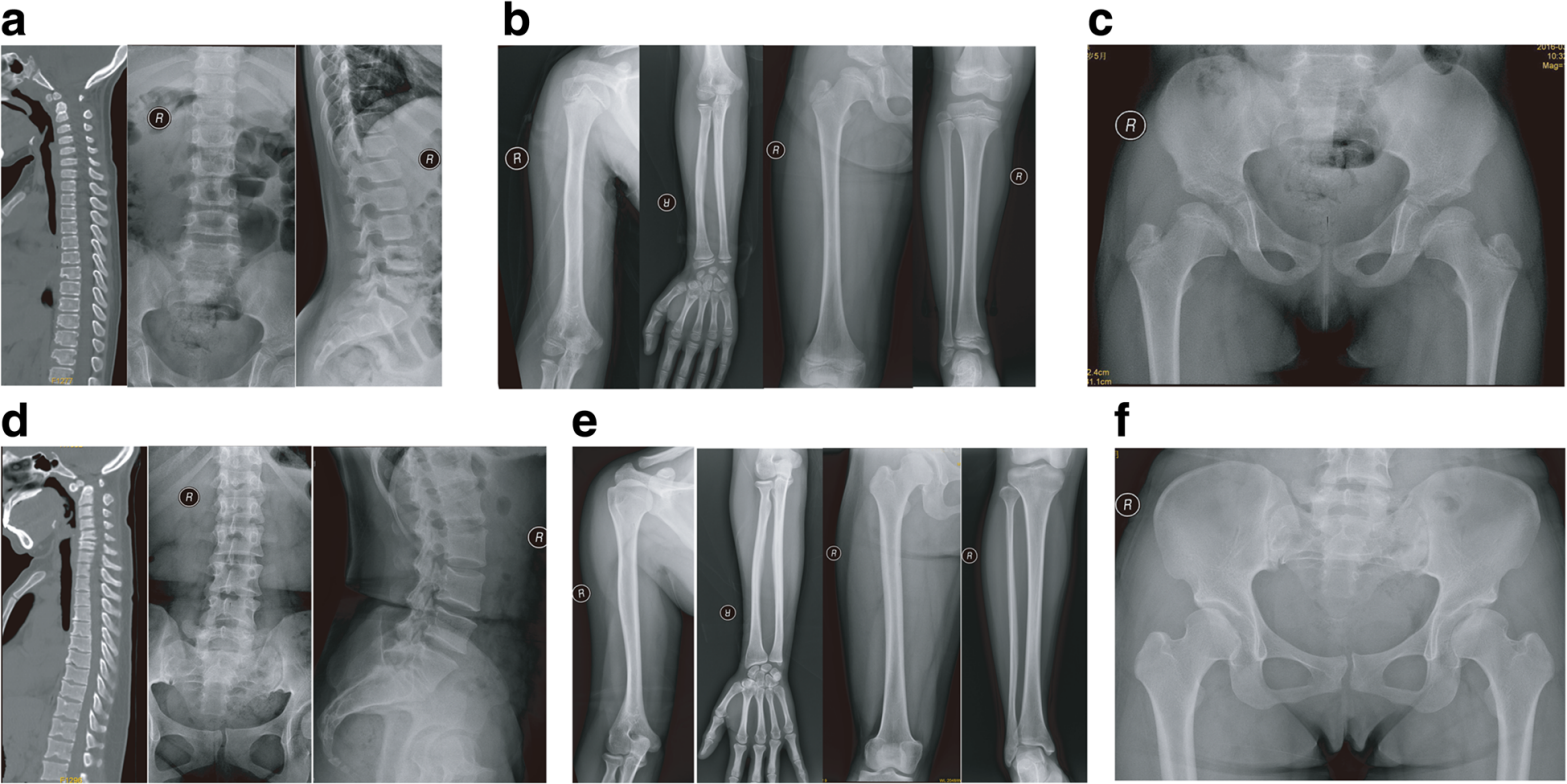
Radiographic findings of patients III-2 and II-3. a Radiographic findings of the spine of patient III-2. The patient displayed platyspondyly (C3–C6) and defective anterior vertebral bodies (T7–T12, particularly at the lower edge). b Radiographic findings of the long bones of patient III-2. Dysplasia was detected in the bilateral femoral heads and distal tibial epiphysis. No particular changes were found in the metaphyses. c Radiographic findings of the pelvis of patient III-2. No particular abnormalities were found in the pelvis. d Radiographic findings of the spine of patient II-3. Os odontoideum and atlantoaxial subluxation were observed in the CT scan of the cervical spine. Other findings included multiple Schmorl’s nodes (T5–T12), platyspondyly (C5–C6, T6–T9), lumbar lordosis, and a marked increase in the lumbosacral angle. e Radiographic findings of the long bones of patient II-3. Dysplasia was found in the bilateral femoral heads and distal tibial epiphysis. No particular changes were found in the metaphyses. f Radiographic findings of the pelvis of patient II-3. No particular abnormalities were found in the pelvis
